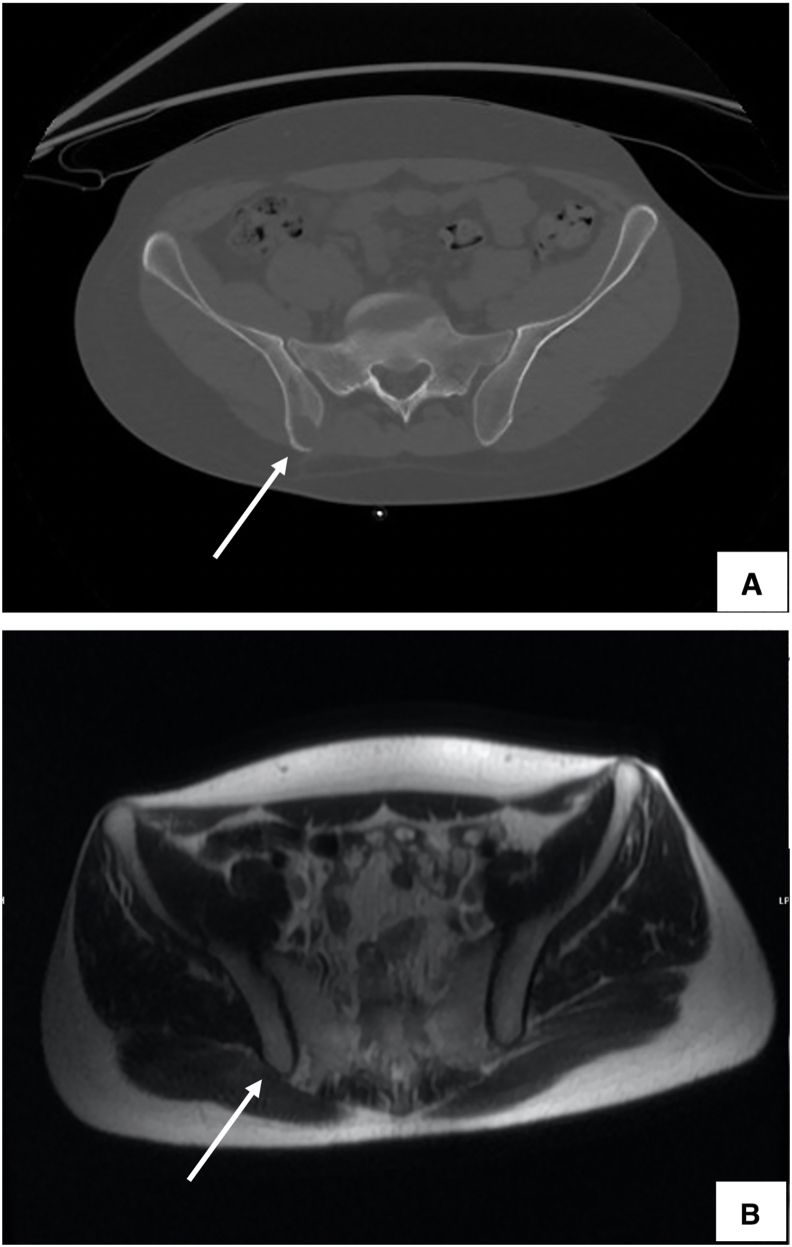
(A) Axial CT pelvis with bone showing a new lytic lesion of the right posterior inferior iliac spine. (B) MRI pelvis with axial SS-FSE sequence 3 years previously showing normal signal intensity in both iliac bones.
